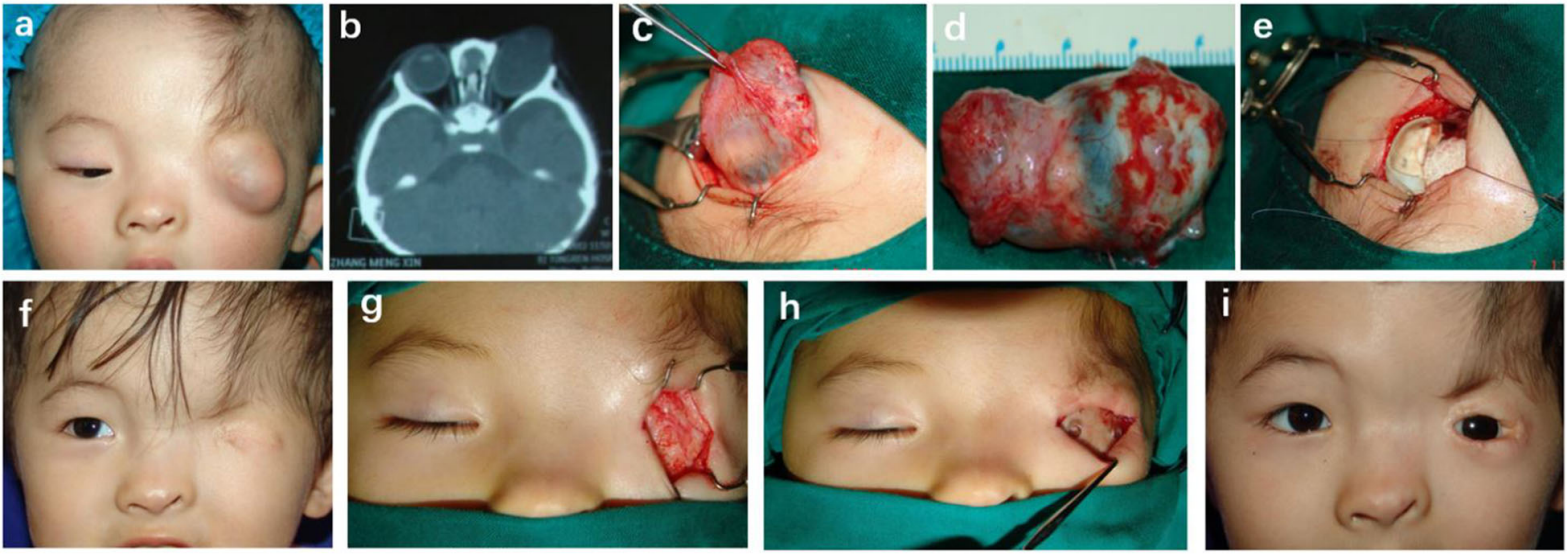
a Preoperative patient with complete cryptophthalmos and a cystic swelling arising from the left orbit. b CT scan shows an enlarged, bulging globe with increased anteroposterior dimensions in the right side. c Intraoperative photograph shows a large cystic globe. d Enucleation of enlarged cystic eyeball. e Implantation of 21.0 mm HA and banked sclera. f One year after the first stage surgery. g Construction of eyelids and socket using full-thickness skin graft. h A plastic eye shell was placed to maintain the conjunctival sac. i 1.5 years after the second stage surgery with prosthesis
